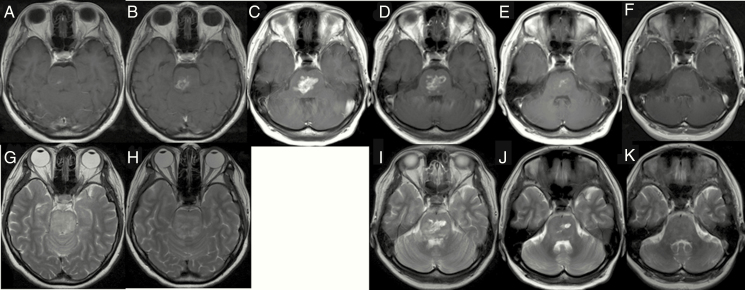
A case of a 32-year-old female treated for DIPG for 4 months prior to starting CED of ACNU. She was treated with local 54 Gy irradiation and then followed up at another hospital for her initial disease (A and G: 3 months before CED). She was referred to us 1 month prior to the CED of ACNU (B and H). Treatment was given against a rapidly enhancing mass (C). After treatment, TMZ monotherapy was continued (D and I) 2 months after CED (E and J: 5 months after CED). Her symptoms of truncal ataxia, diplopia, and dysarthria gradually recovered. Imaging revealed complete remission of the tumor at 7 months after the CED of ACNU (F and K). (A–F) T1-weighted images with contrast enhancement and (G–K) T2-weighted images.
